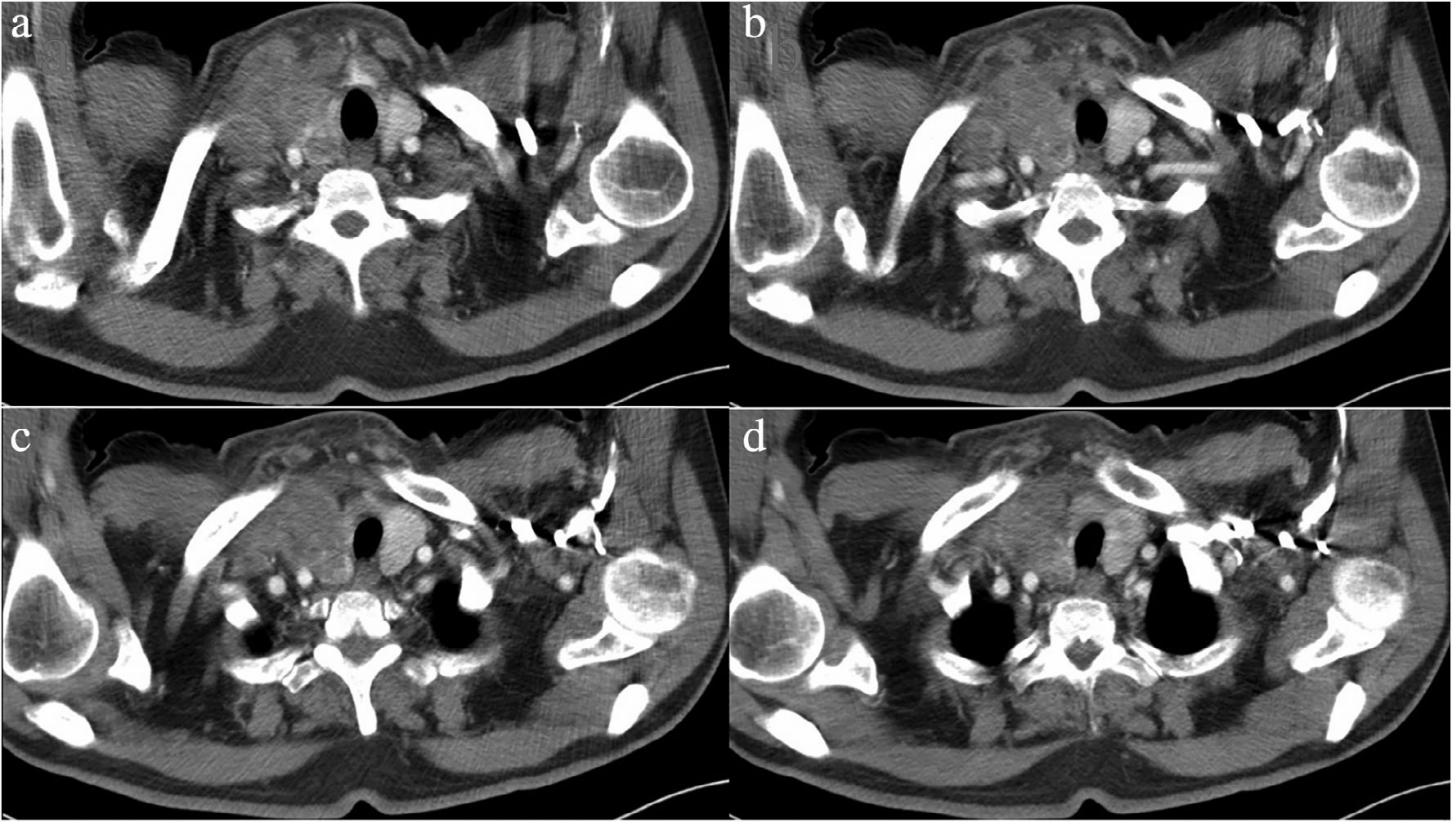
Cervical computed tomography (CT) scan revealed a 4.5 × 3.7 cm mass in the right thyroid gland of patient No. 3.
